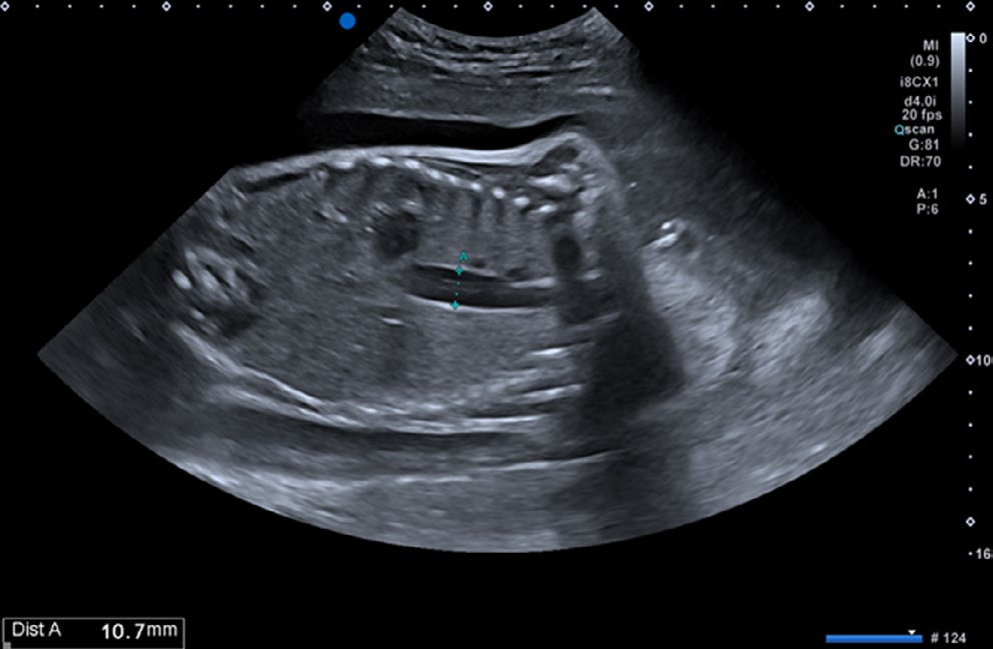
Prenatal ultrasonography showed a dilated esophagus and gastric bubble.
